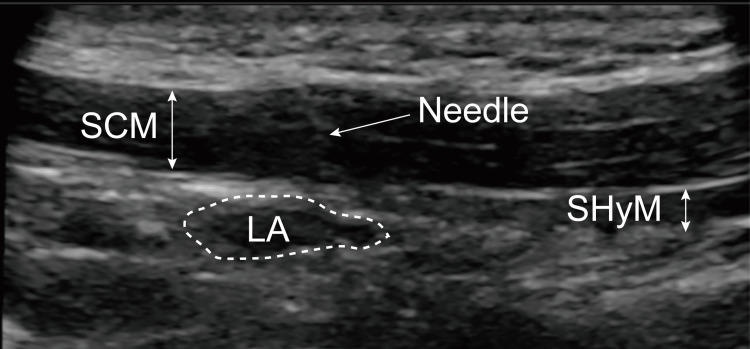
Ultrasound image of the UGSGNB. The sternocleidomastoid muscle and the stylohyoid muscle can be observed behind the mandibular ramus. We confirmed the spread of the local anesthetic. SCM, sternocleidomastoid muscle; SHyM, stylohyoid muscle; LA, local anesthetic; UGSGNB, ultrasound-guided selective glossopharyngeal nerve block.
